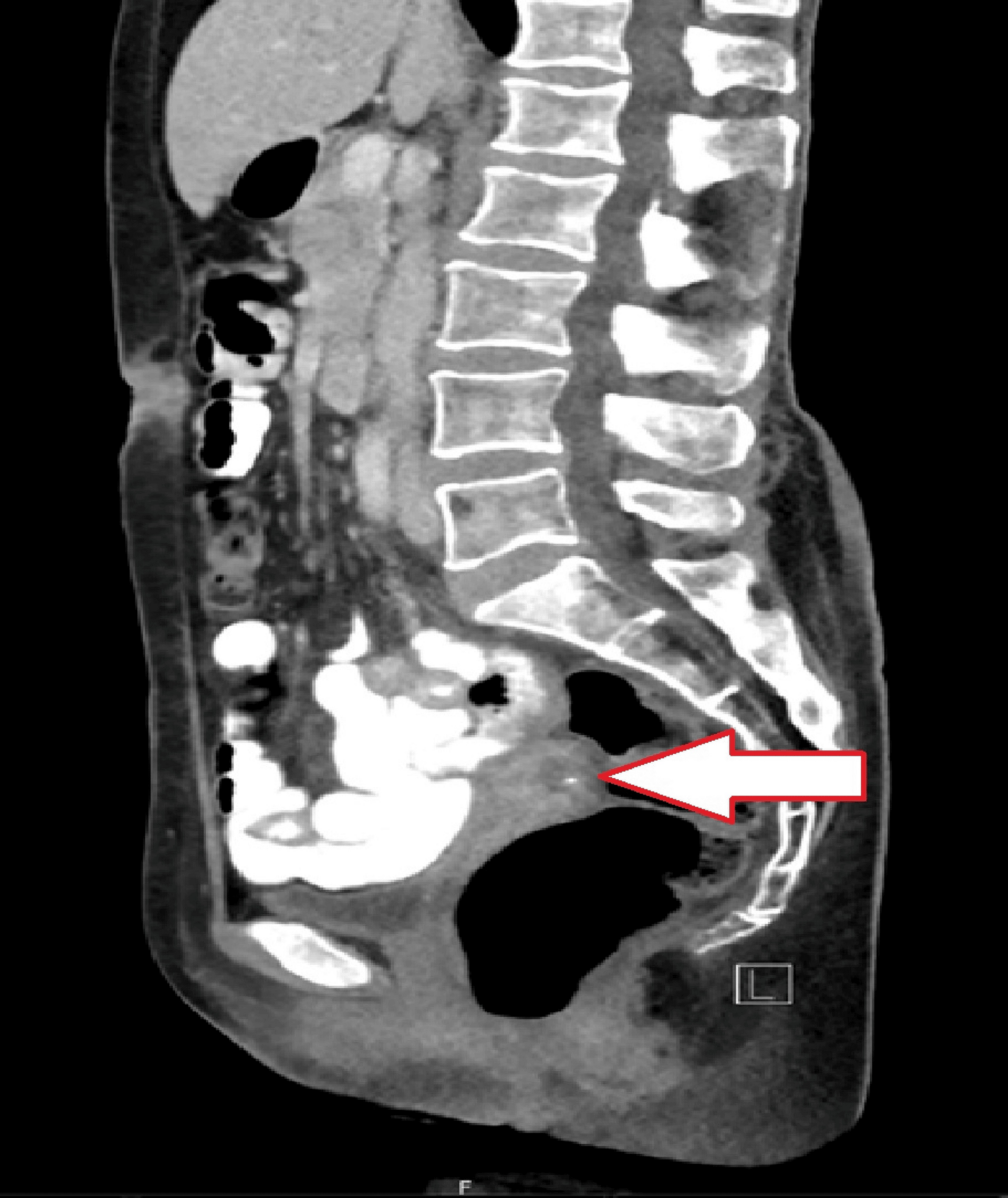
Left of the midline, sagittal cut of CT abdomen/pelvis showing a fluid collection
